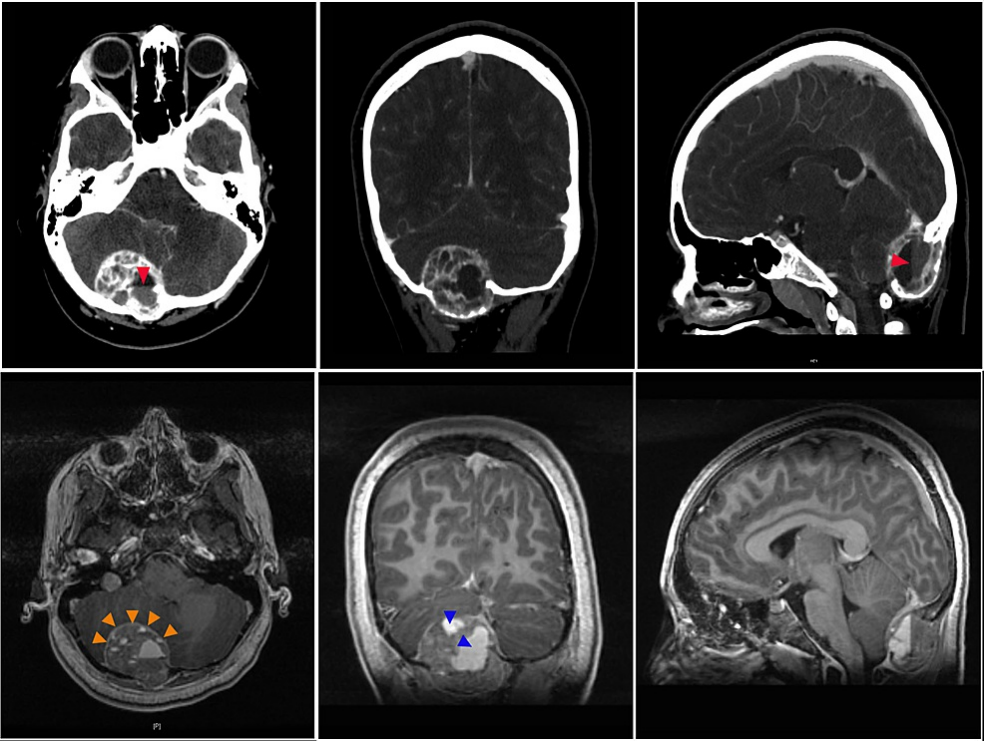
CT and MRI Brain Demonstrating Giant Cell Tumor of Right Occipital Bone Computed tomography and magnetic resonance imaging with contrast (top and bottom rows, respectively) demonstrate a 3.6 x 4.7 x 3.4 cm expansile lesion arising from the diploic spaces of the right occipital bone, expanding both inner and outer cortex and containing multiple loculations with blood-fluid levels (red arrowhead). Solid, soft tissue components avidly enhance with contrast (green arrowhead). There is mass effect on the right cerebellum without parenchymal involvement (a clear cerebral spinal fluid plane is visible, orange arrowhead) and mild effacement of the fourth ventricle without ventriculomegaly. The lesion extends to the inferior margin of the torcula and abuts the sagittal and proximal right transverse sinuses without stenosis or invasion.
